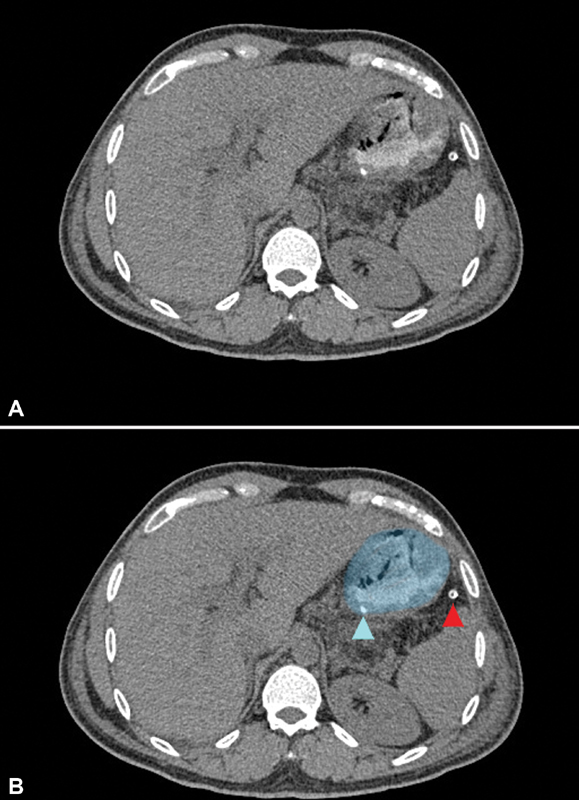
( A ) A postoperative computed tomography (CT) scan with luminal
